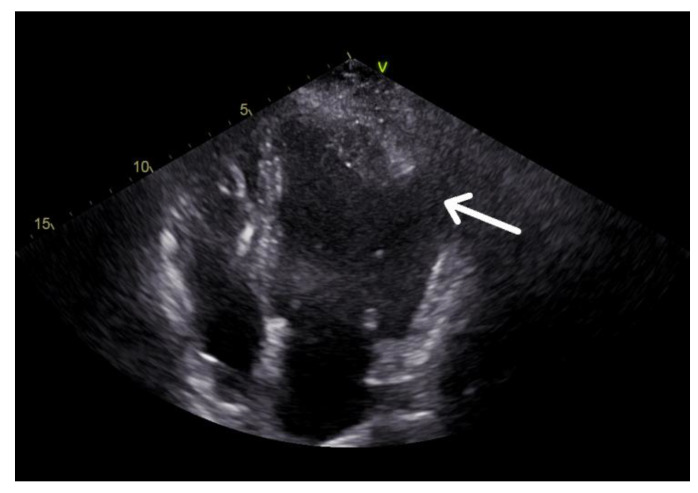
Two-dimensional transthoracic echocardiography (TTE) of a 4-chamber apical view shows an enlarged hypokinetic left ventricle. At the level of the apex, there was something resembling a left double-chambered ventricle (DCV, arrow).
